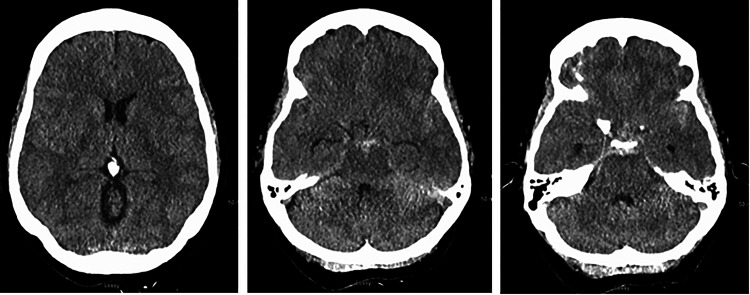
A 42-year-old female (patient 3) presenting with sudden onset of headache and mild confusion. Axial view of the noncontrast head CT showing (a) no signs of obstructive hydrocephalus, (b) SAH in the interpeduncular cistern and bilateral sylvian cisterns, and (c) SAH in the prepontine cistern. CT: computed tomography; SAH: subarachnoid hemorrhage.
